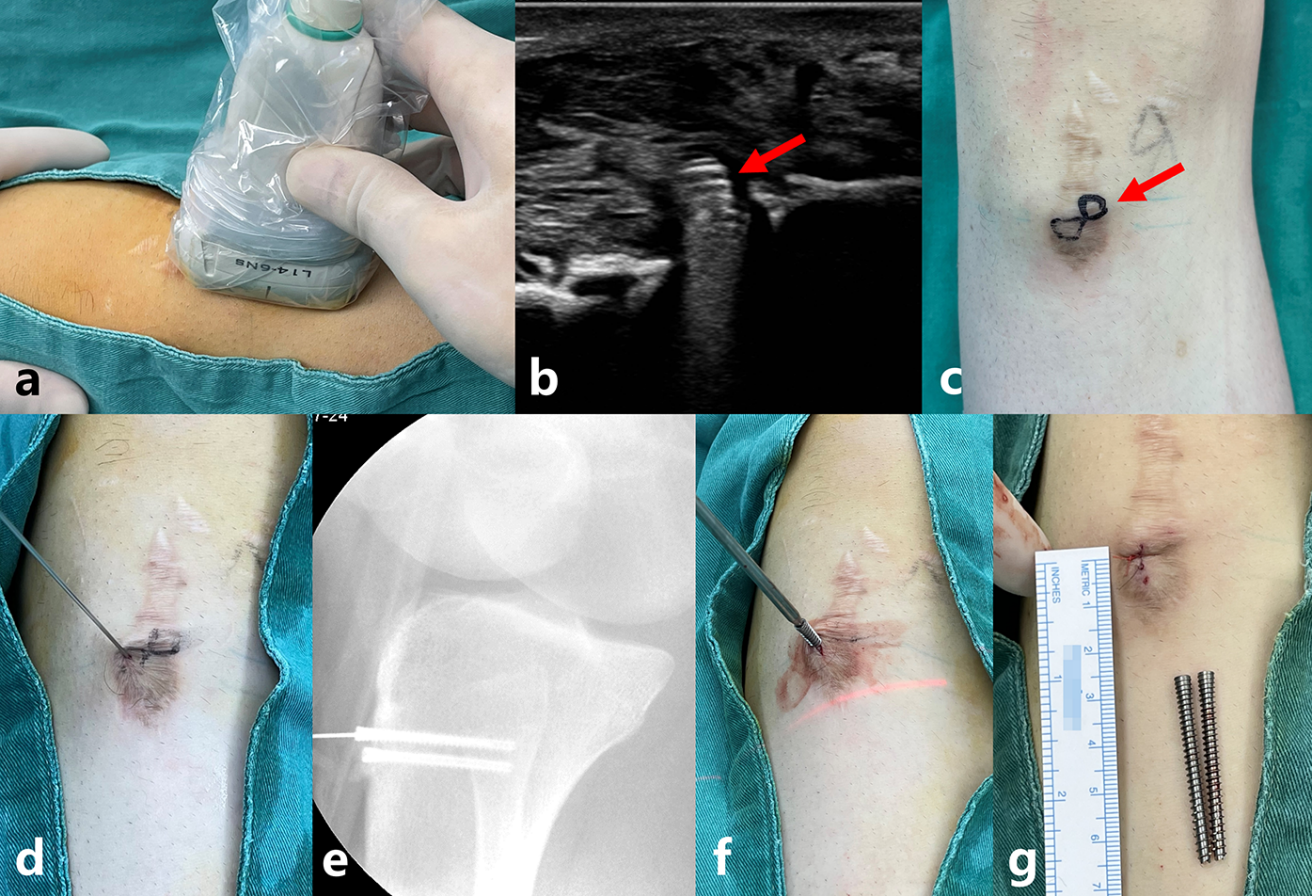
Shows a typical patient. A 13-year-old boy who came to remove the cannulated screws 14 months after right tibial tuberosity fracture surgery. He was injured because he tripped while running. (a) shows the position of the probe on the body surface. (b) shows the ultrasound image of the screw. The red arrow shows the screw tail. (c) shows the surface position of the screws. The red arrow shows the mark of the screw tail on the skin. (d) shows the penetration of the guide needle into the cannulated screw. (e) shows a radiograph confirming that the guide needle is inside the cannulated screw. Radiographs are not required after becoming proficient in surgical operations. (f) shows the placement of the screwdriver along the guide needle and the removal of the screw. (g) shows the incision being only 0.5 cm, and the screws were successfully removed.
